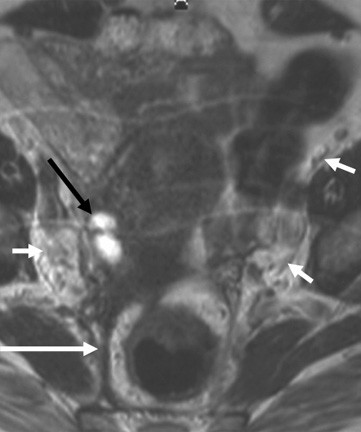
MRI axial T2W image of the pelvis following treatment for sarcoidosis. The left adnexal cyst has resolved. There is a small residual cyst on the right (black arrow). There is marked decrease in the thickening of the mesorectal fascia (white arrow). The lymph nodes appear normal (short white arrows).
